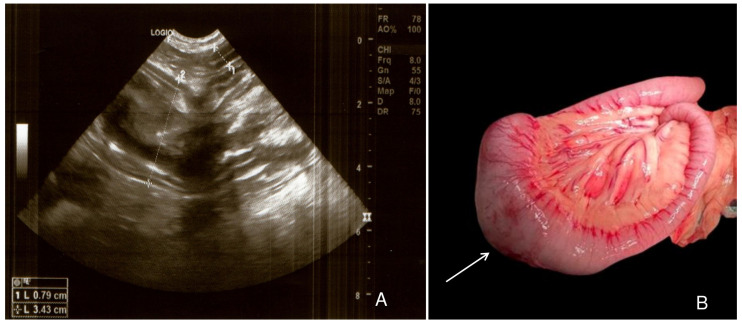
Ultrasound and macroscopical features of the canine intestinal osteosarcoma. The mass is characterized by a hypoechoic external region and an anechoic center (measured area 2); in the top right corner there is a normal intestinal loop (measured area 1); (A). Grossly, the intestinal wall is severely distended by a poorly delimited, infiltrative and dense mass (white arrow) (B).
